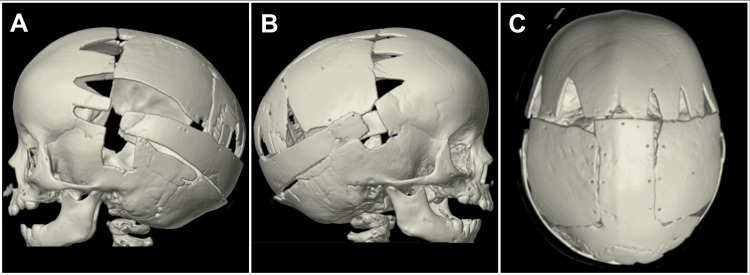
Post-surgical CT 3D reconstruction Demonstrating the modified Melbourne procedure. Our modifications include using a more formal fronto-orbital remodeling technique rather than the simple barrel-staving of the "F" flap. We also use morsellized bone paste "salami" to fill all bone defects during the surgery. CT: computed tomography, 3D: three dimensional
